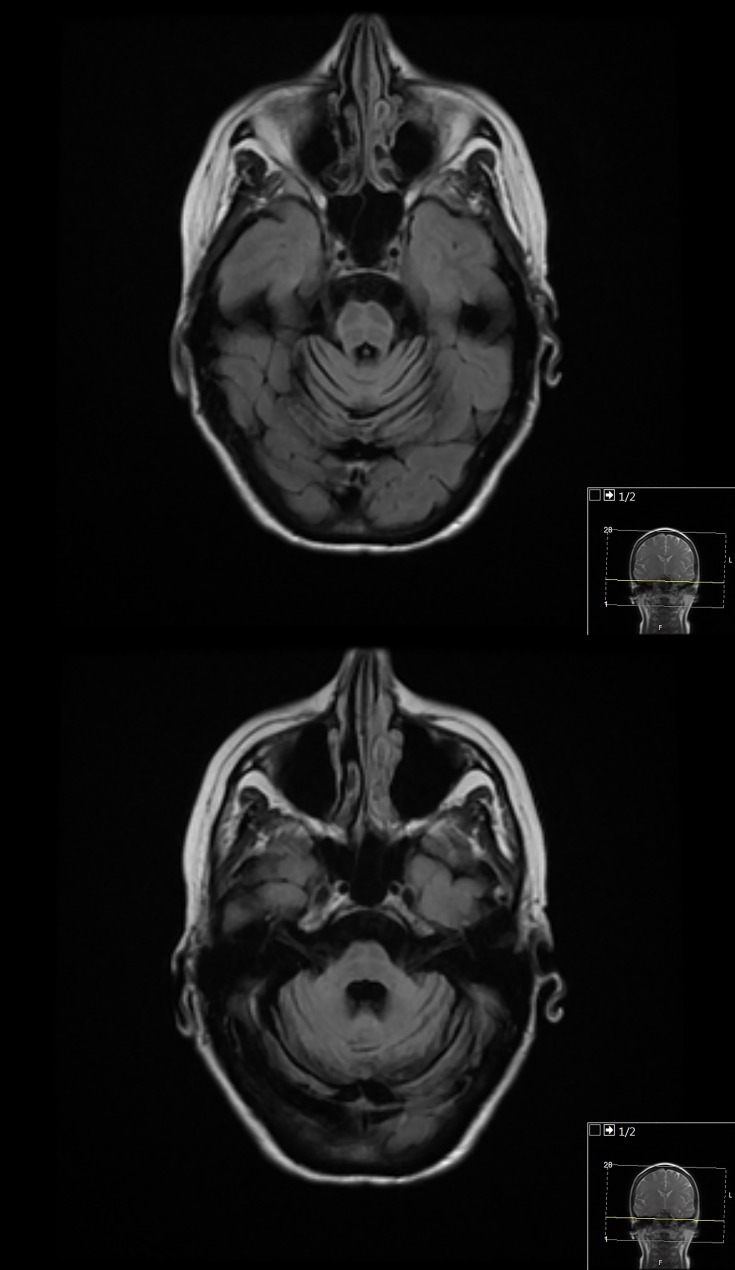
Fluid-attenuated inversion recovery (FLAIR) axial image of the brain, showing pontine and cerebellar atrophy, and ‘hot-cross bun’ sign, in a patient with multiple systems atrophy type C (MSA-C). Note the narrow middle cerebellar peduncles.
